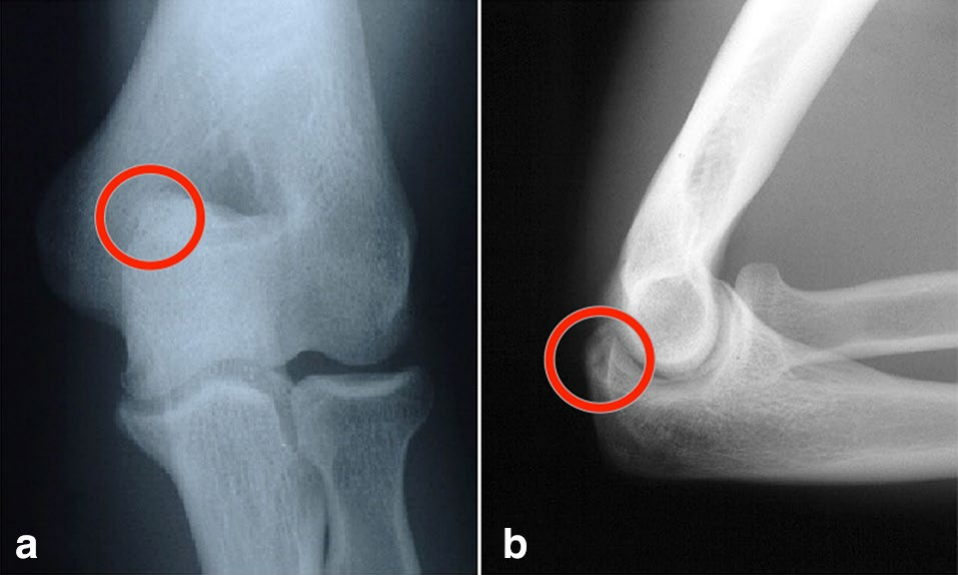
Preoperative radiographs with a circle demonstrating the olecranon osteophyte. a The preoperative AP radiograph showing posteromedial olecranon osteophytes. b The preoperative lateral radiograph showing posterior olecranon osteophytes
